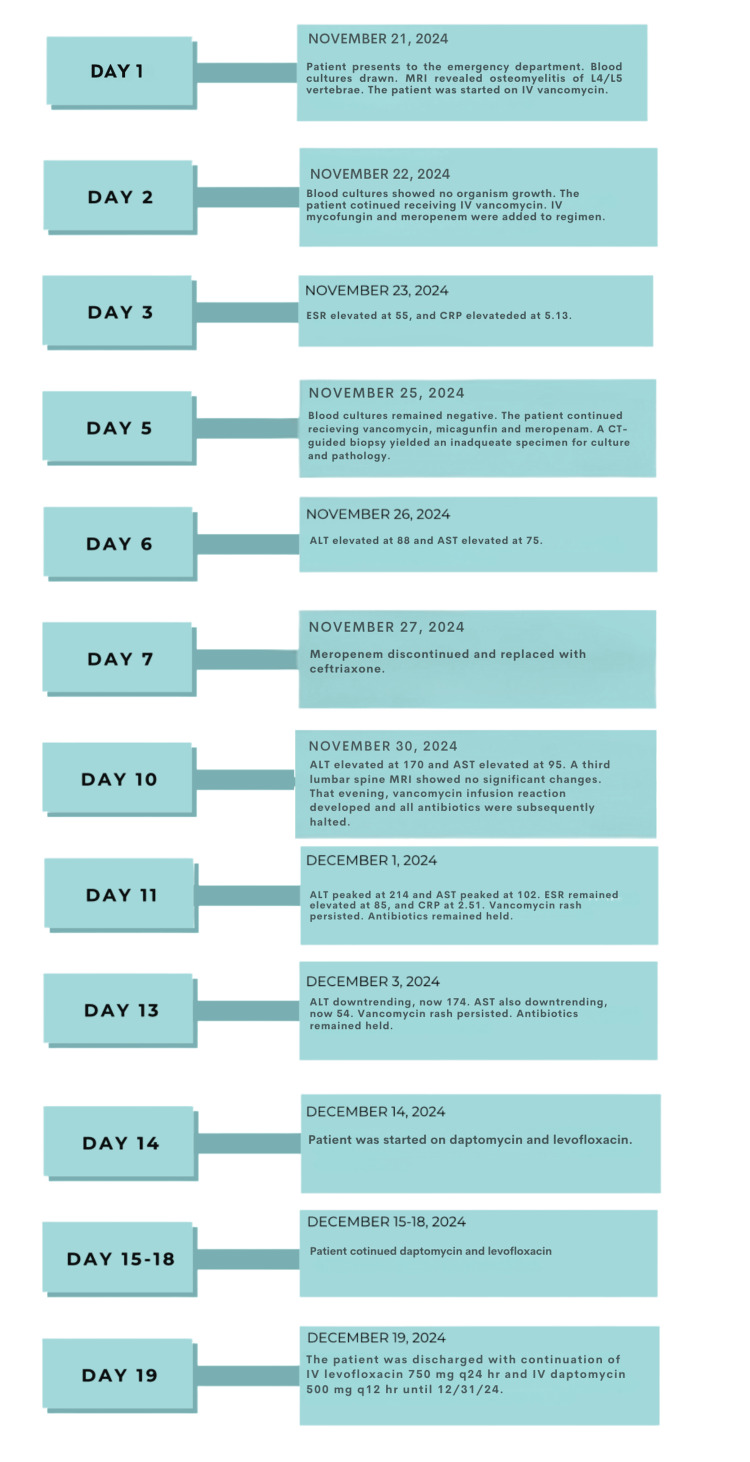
Timeline of hospital course and management MRI: magnetic resonance imaging; IV: intravenous; ESR: erythrocyte sedimentation rate; CRP: C-reactive protein; CT: computed tomography; ALT: alanine aminotransferase; AST: aspartate aminotransferase; mg: milligrams; q24 hr: every 24 hours; q12 hr: every 12 hours
